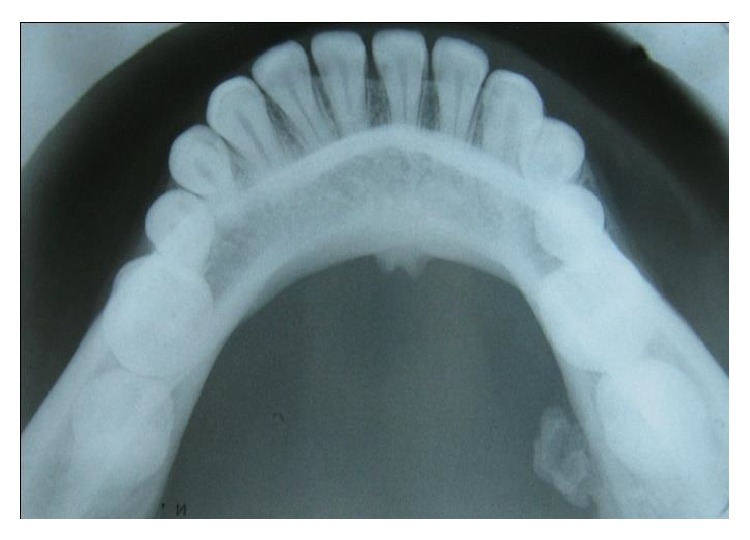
Mandibular occlusal cross-sectional radiograph.
